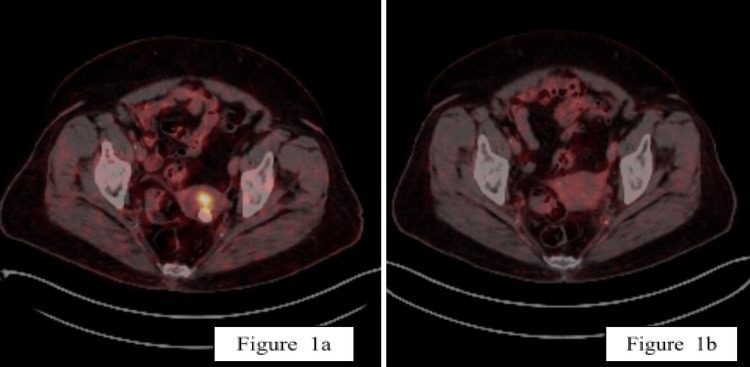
PET-CT scans prior to treatment and after treatment. (a) PET-CT scan before treatment. (b) PET-CT scan three months after treatment showing complete metabolic response. PET-CT = positron emission tomography-computed tomography
